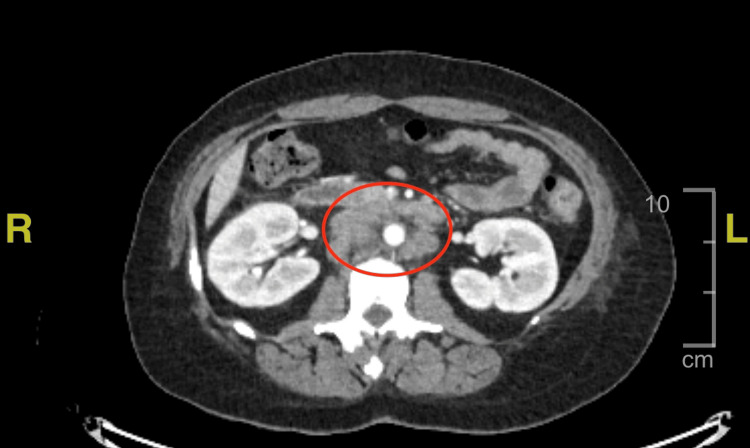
Computed tomography (CT) scan of the abdomen/pelvis with contrast demonstrating numerous enlarged periaortic lymph nodes Red oval: multiple periaortic lymph nodes of varying sizes
